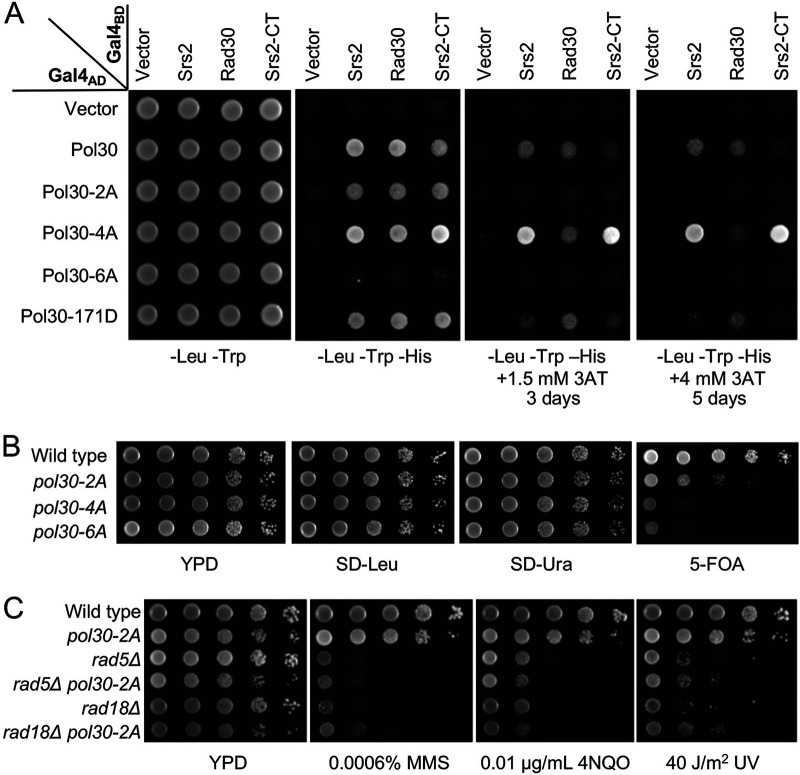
Phenotypes of Pol30 IDCL and C-terminal domain mutations. (A) Physical interaction between Pol30 mutations and Srs2 by a Y2H assay. Rad30 served as a reference. *SRS2*, *SRS2-CT* and *RAD30* were cloned into pGBT9 (Gal4_BD_) and Pol30 mutations that disrupt either IDCL (L126A, I128A = 2A) or the C terminus (252-255AAAA = 4A), or both (=6A) were cloned into pGAD424 (Gal4_AD_). Experimental conditions were as described in Fig. 3. (B) The viability assay for *pol30* mutants after plasmid shuffling. WXY939 cells transformed with YCpL-Pol30 derived plasmids were grown on YPD overnight to allow plasmid loss. Cells were then subjected to a serial dilution by spotting on SD-Leu to select those carrying YCpL-Pol30 based plasmid, on SD-Ura to select those carrying pBL211 (YCpU-POL30), and on 5-FOA to select cells that had lost the pBL211 plasmid. (C) Phenotypes of *pol30-2A* in rescuing *rad5Δ* or *rad18Δ* mutants from killing by DNA-damaging agents in a serial dilution assay. Experimental conditions were as described in Fig. 2. All plates were incubated at 30°C for 2 days before photography.
